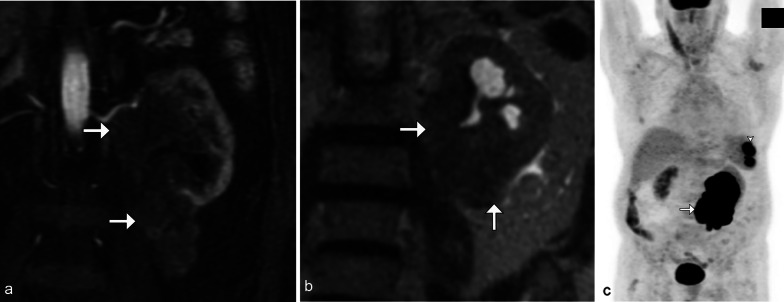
Renal sinus involvement of renal lymphoma.* Coronal MRI post contrast (a) and T2 weighted (b) images demonstrate a T2 hypointense, hypoenhancing infiltrative left renal mass (arrows) invading the upper pole collecting system and lower kidney. Note the resultant mild hydronephrosis relative to tumor size. PET/CT (c) shows the marked F18-FDG avid left renal mass (arrow) as well as splenic masses (arrowhead). This was biopsy-proven high-grade B-cell lymphoma
